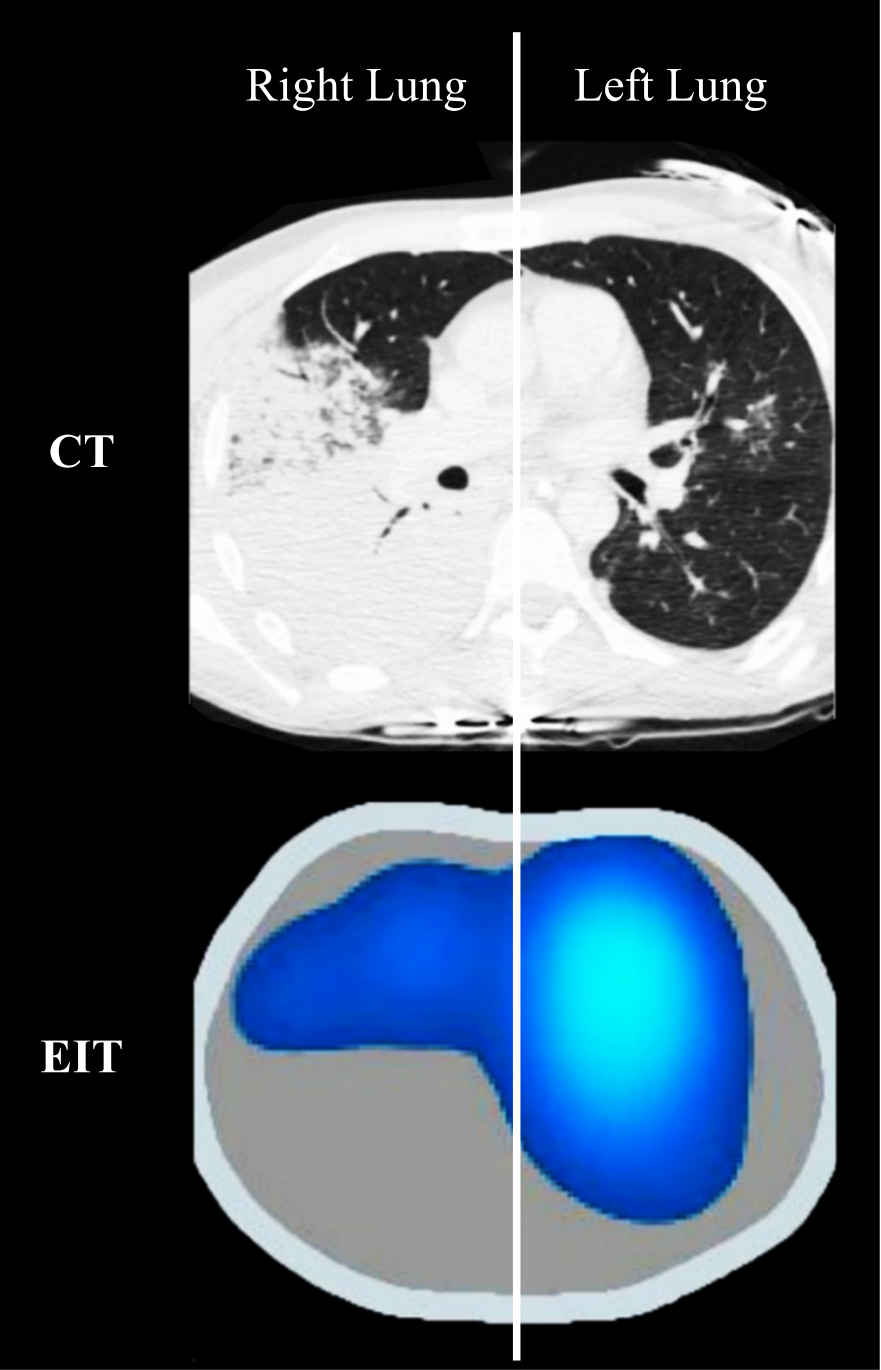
Computed tomography (CT) of a patient with pneumonia and corresponding functional image obtained from electrical impedance tomography (EIT). Note absence of ventilation on lower right lung in EIT image and corresponding massive consolidation on right lung assessed by CT
